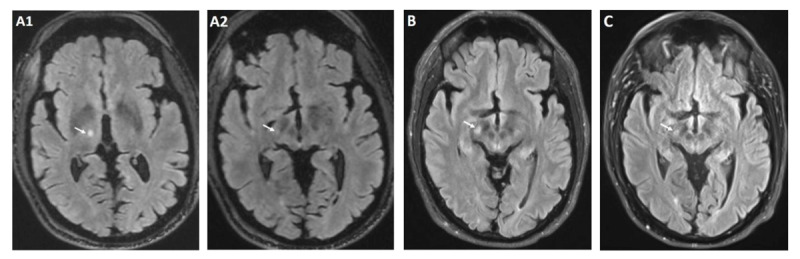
Brain MRI T2 FLAIR post procedure. Immediately post procedure lesion at Vim (A1) with very slight extension to STN (A2) 1 month after procedure and onset of chorea with increased signal intensity involving the right STN (B) 8 months after procedure with near resolution of STN signal hyperintensity (C).
